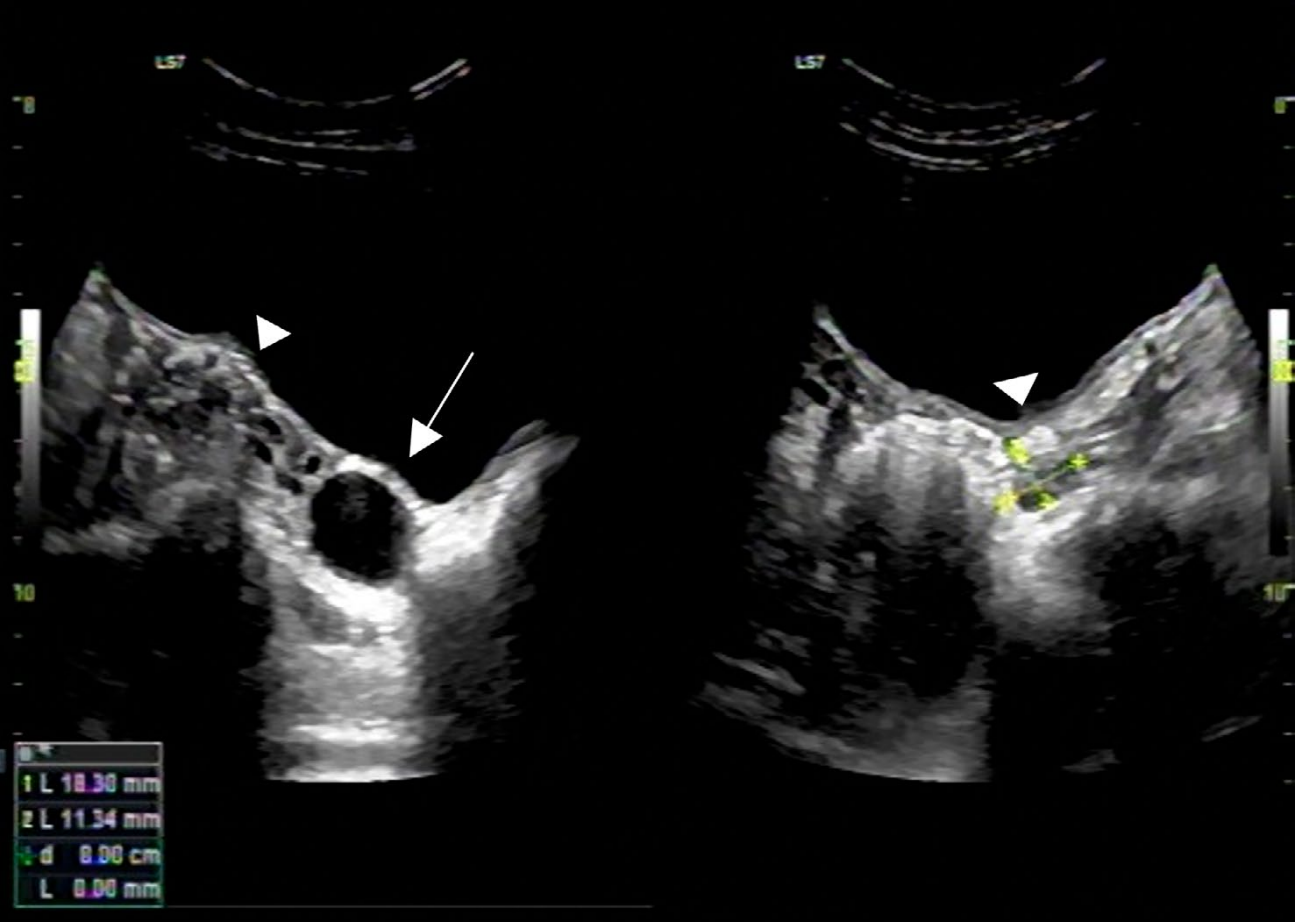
Ultrasound characteristics of a mass in the right ovary. A well‐circumscribed, anechoic cystic mass, measuring approximately 28 × 24 × 25 mm (solid arrow). The right ovary (arrowhead) measured 19 × 17 mm; the left ovary (arrowhead) measured 18 × 11 mm.
